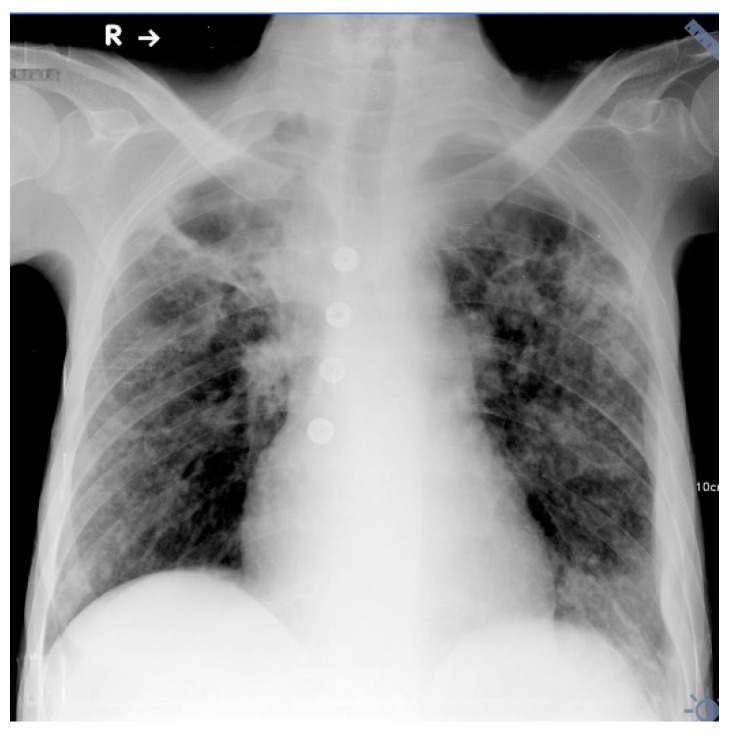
Chest X-ray anteroposterior in a horizontal position. Extensive, parenchymal consolidations in both lungs with low-attenuation areas in upper zones suggest cavitations.
